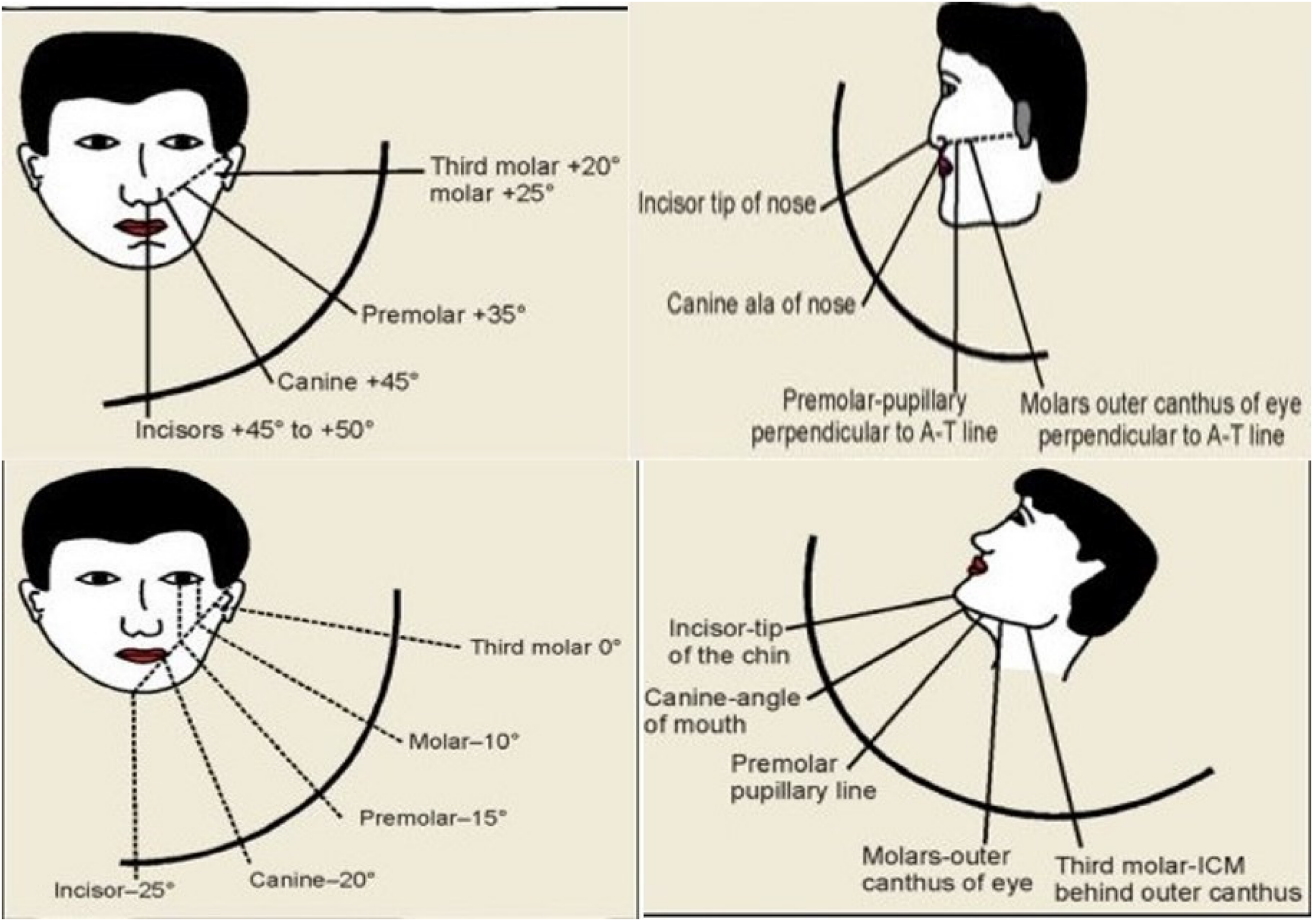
Standard conventional radiographic reference points for maxillary and mandibular teeth periapical projections.
